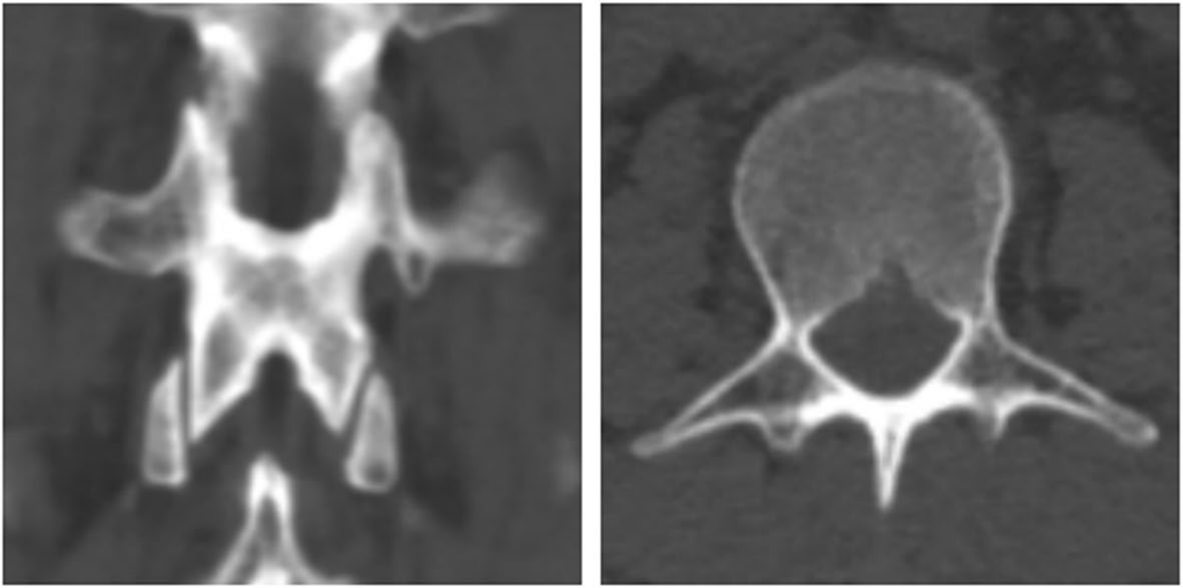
No laminar fractures. CT axial and coronal planes show no laminar fractures
